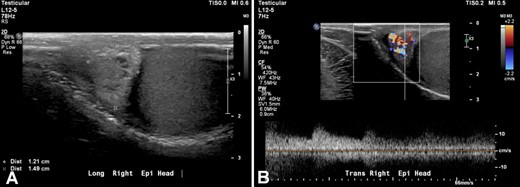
Color flow and spectral doppler of the right epididymis in sagittal plane. Results demonstrated: A – a right paratesticular (epididymal) mass (1.49 cm in greatest dimension); B – with increased vascularity.
